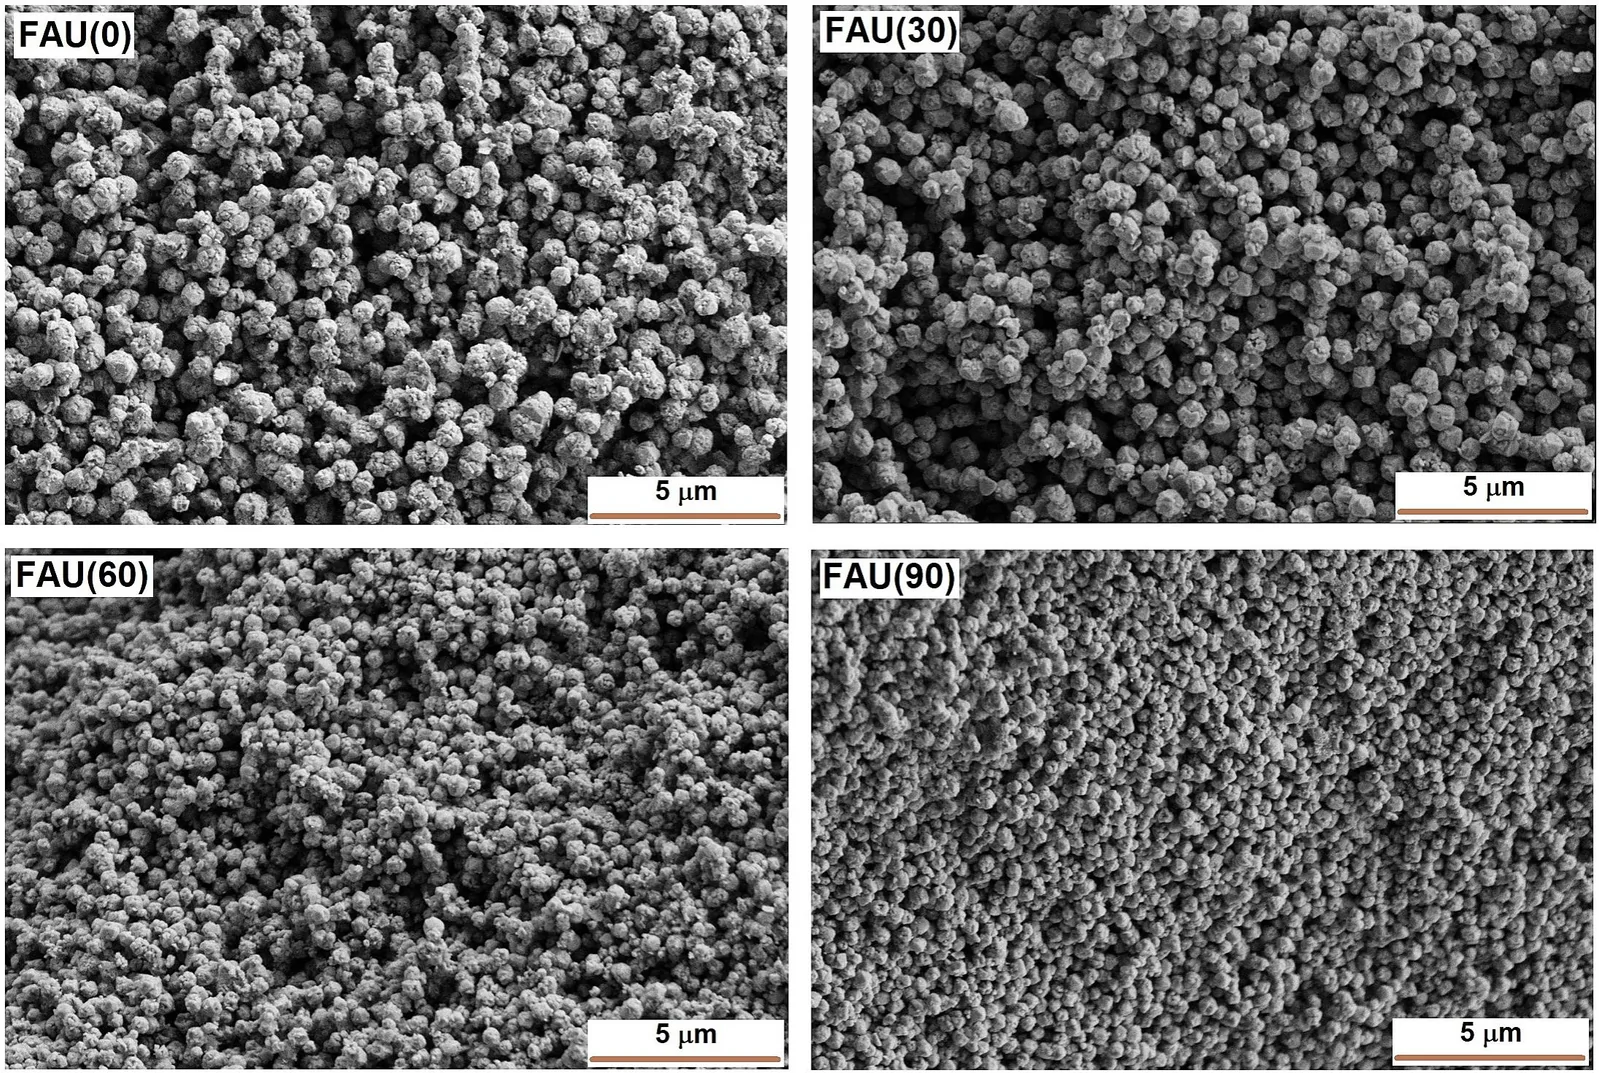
SEM images of the samples under study (magnification 20,000x). The influence of the exposure time of zeolite gel to ultrasonic irradiation on the morphology of the prepared zeolite systems.
